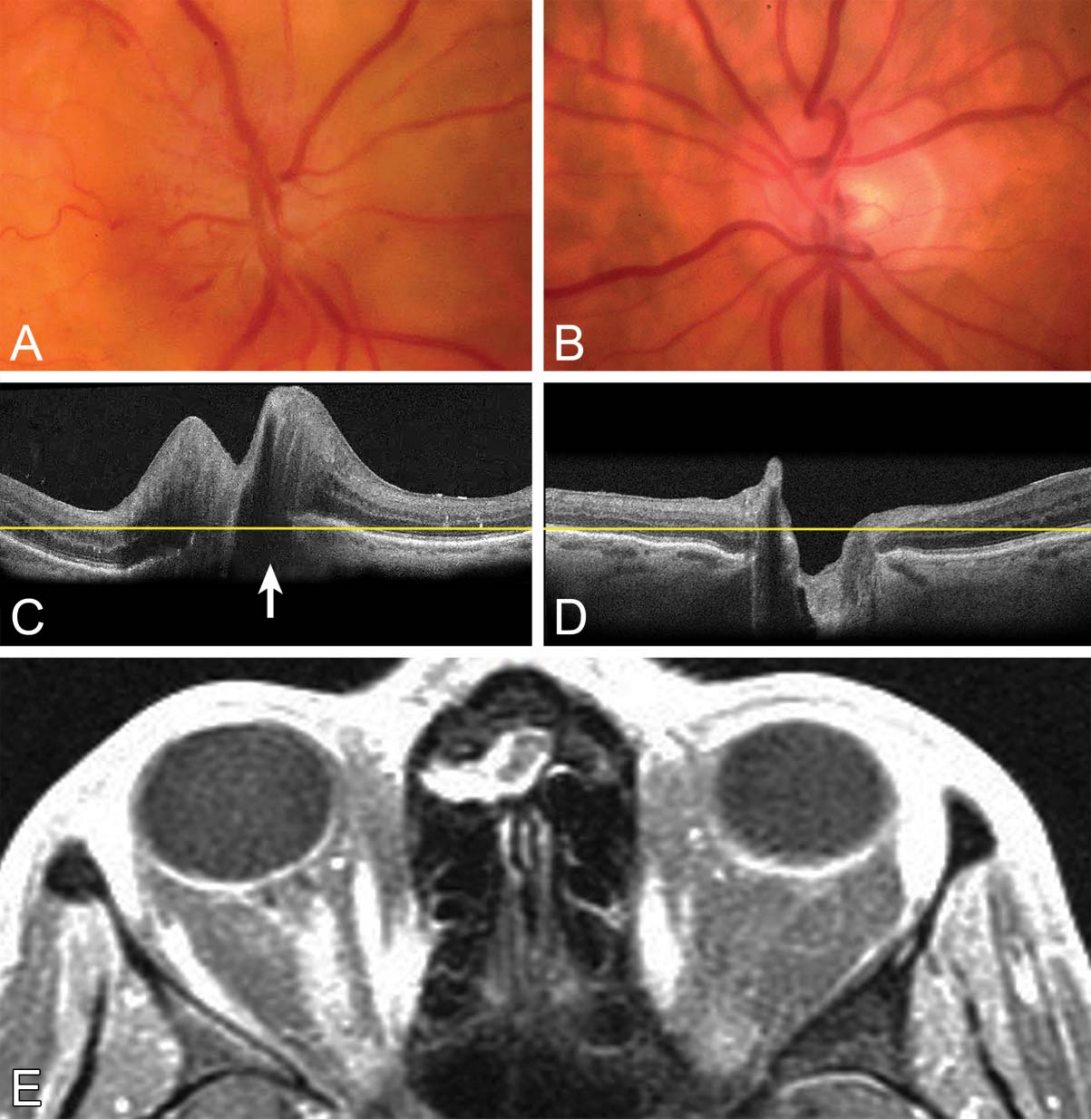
A 66-year-old diabetic woman with sudden painless vision loss, 20/40, an afferent pupillary defect, a nasal step, and unilateral optic disc edema in the right eye (A). The left eye was normal with cup-to-disc ratio of 0.2 (B). A presumptive diagnosis of nonarteritic anterior ischemic optic neuropathy was made. Because the transverse axial OCT showed anterior deformation with a W-shape in the right eye (C) vs V-shape in the left eye (D), we obtained an MRI (E) that showed a presumed optic nerve sheath meningioma. OCT, optical coherence tomography. Yellow line is a reference that joins the peripheral margins of Bruch's membrane layer. The white arrow highlights the anterior displacement or BML relative to the yellow reference line.
